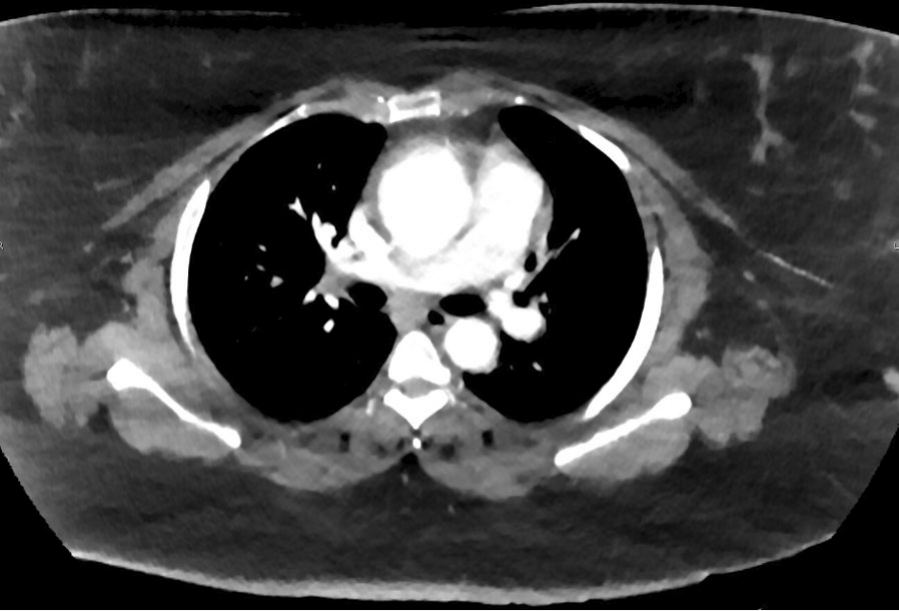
Computed tomogram of an ascending aortic aneurysm with a suspected intramural hematoma
